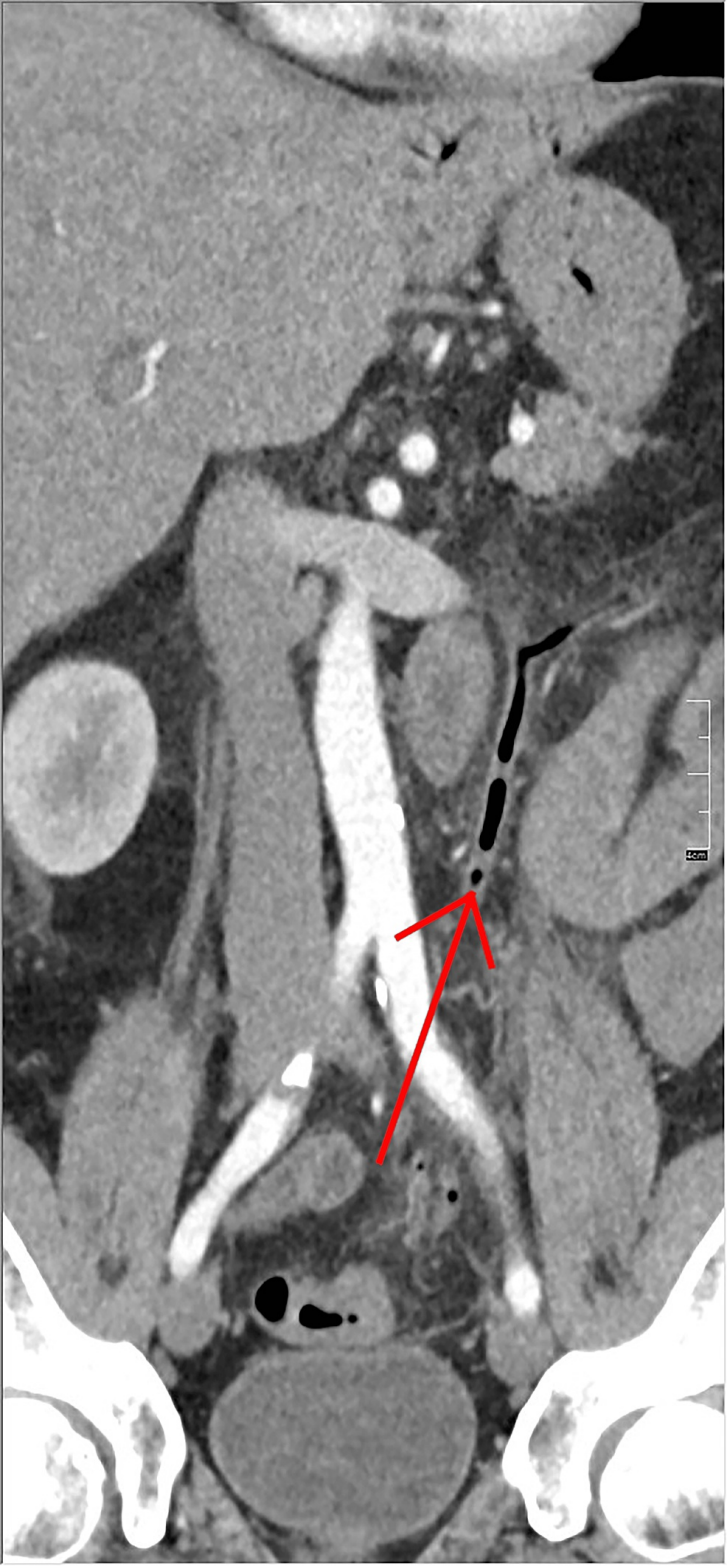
Abdominal CT scan with an injection of contrast showing thrombophlebitis and air within the inferior mesenteric vein. CT, computed tomography
